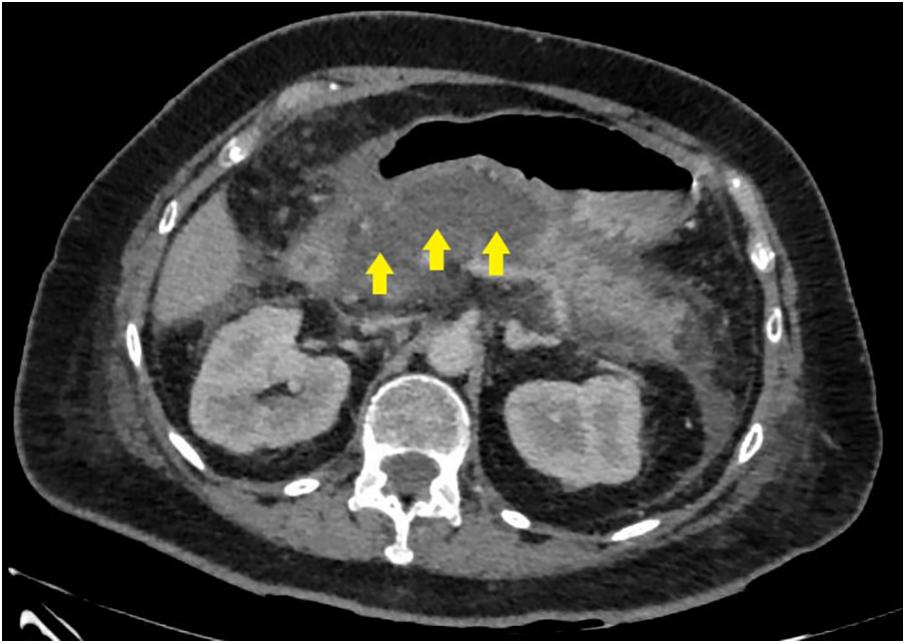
CT image before endoscopic transpapillary stent placement in the main pancreatic duct in patients with necrotizing pancreatitis (stents in the main pancreatic duct and common hepatic duct are indicated by arrows).
